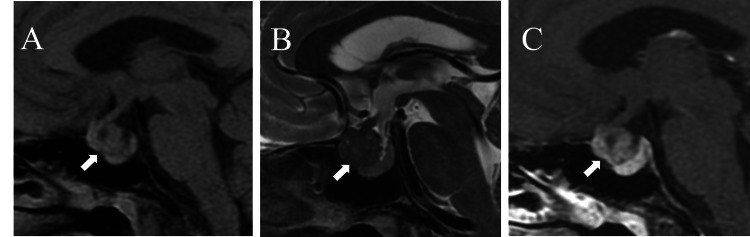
Preoperative MRI of the pituitary gland (A) Sagittal position on T1WI, a round-like isointense shadow could be observed in the suprasellar region; (B) sagittal position on T2WI, a round-like low-signal shadow can be seen; (C) sagittal position of T1 contrast enhancement. A round-like soft tissue mass was observed in the suprasellar region with mild enhancement (white arrow), clear boundary, and pituitary gland located at the posterior and inferior parts of the lesion
